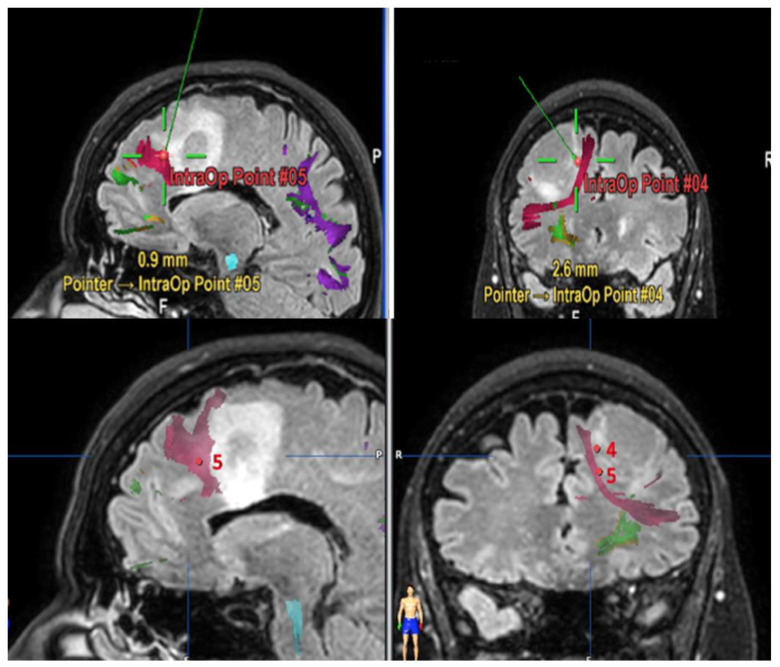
Intraoperative screenshots of FLAIR MRI sequences with navigated tractography of left frontal low-grade glioma. Point 4 corresponds to the stimulation of the medial part and the point 5 to the middle part of FAT.
